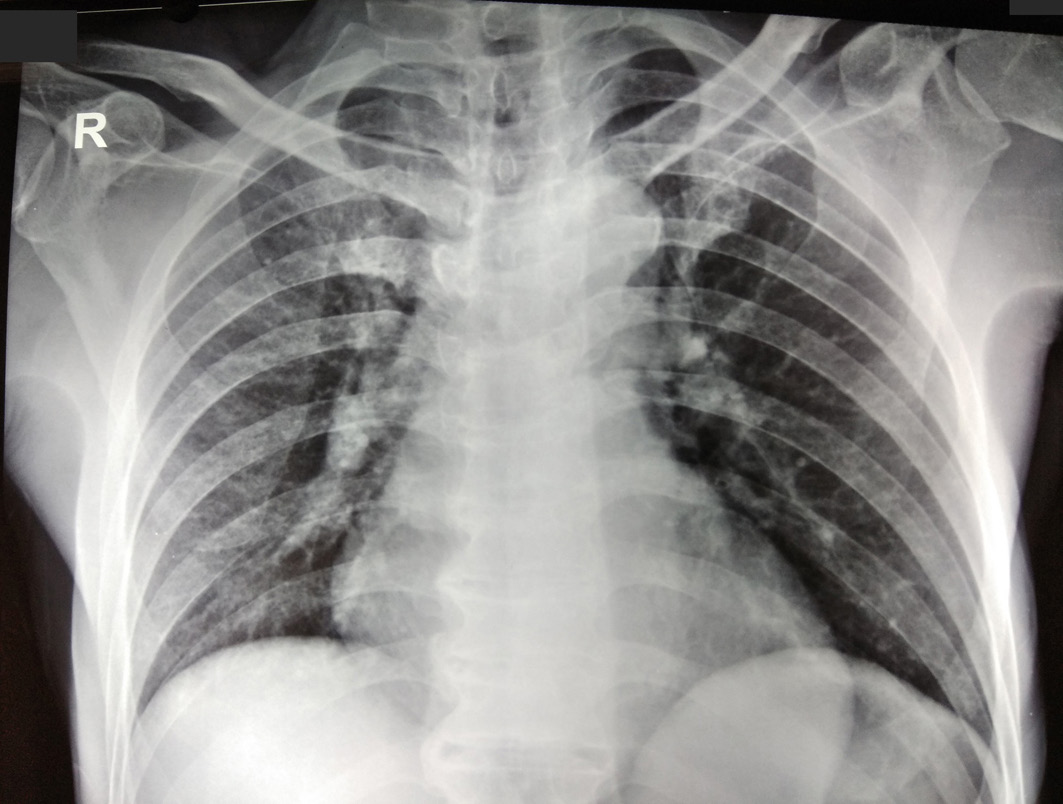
Chest X-ray anteroposterior view showed no abnormalities with cardiothoracic ratio of 47%.
